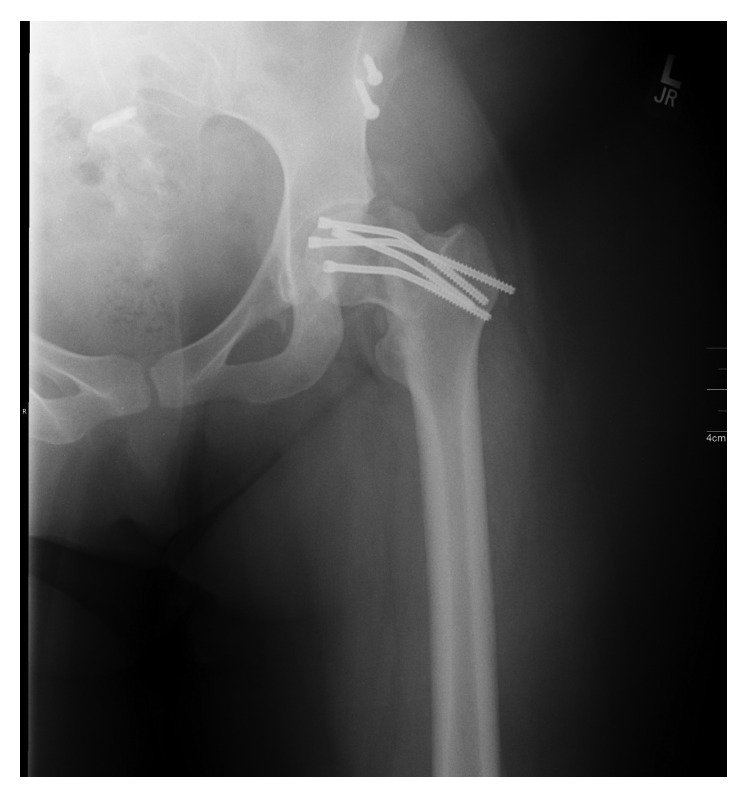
Anteroposterior radiograph showing bending of screws and hardware failure.
